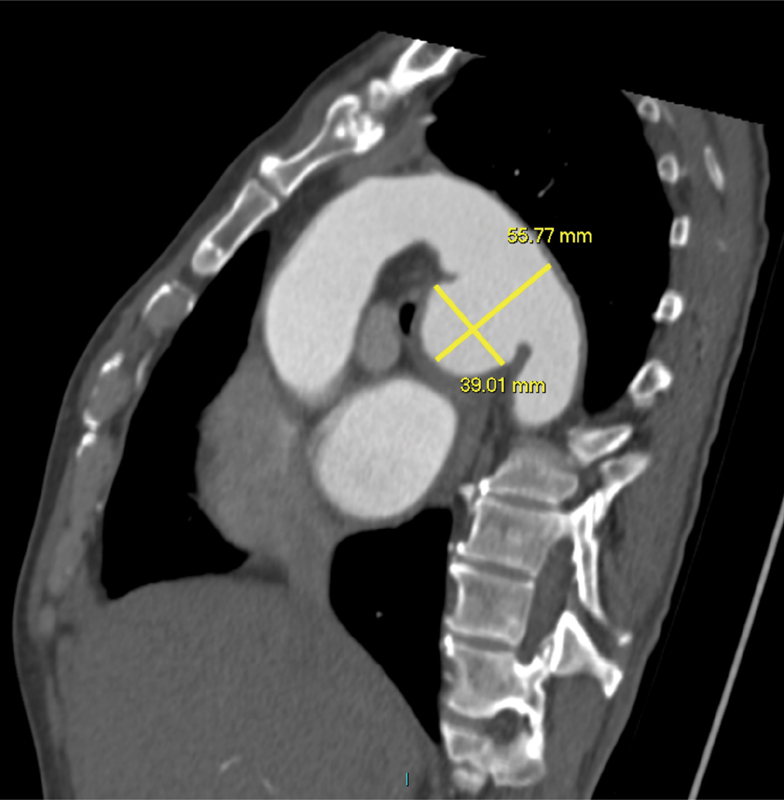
Sagittal section of a contrast-enhanced computed tomography depicting an anterior saccular aneurysm of the descending thoracic aorta with a maximum caliber of 56 mm.
